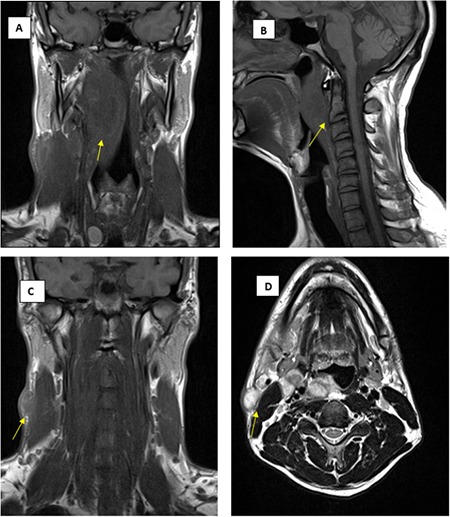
A,B) Magnetic resonance images of the retropharyngeal abscess occluding the right oropharynx (yellow arrows). C,D) Magnetic resonance images of lymphadenopathies including cystic and necrotic areas in the right submandibular region (yellow arrows)
